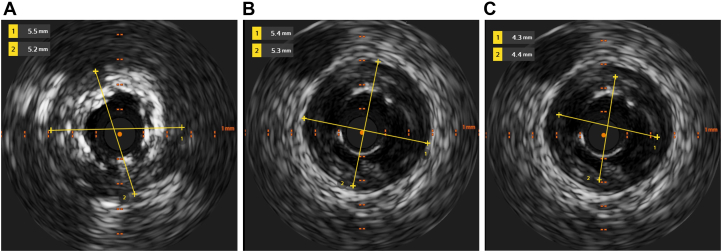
Intravascular Ultrasound Images (A) Proximal vessel diameter, (B) distal vessel diameter, and (C) lumen diameter.
